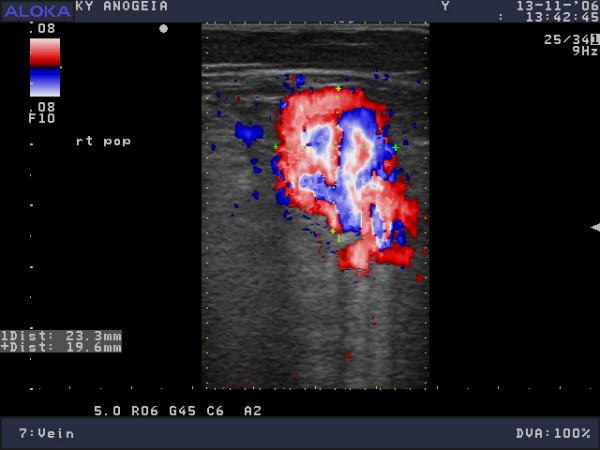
Distal right popliteal vein, colour Doppler ultrasonogram, oblique-transverse axis.
